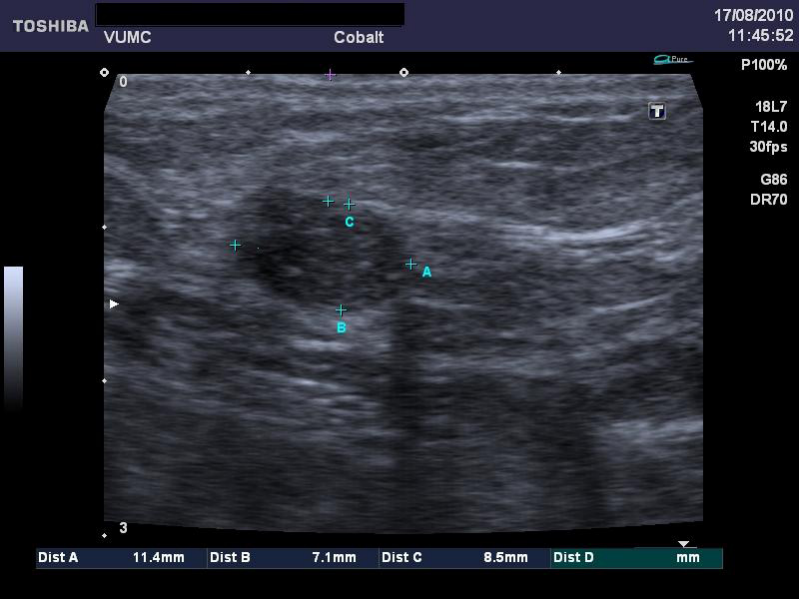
Ultrasound image on screen.
